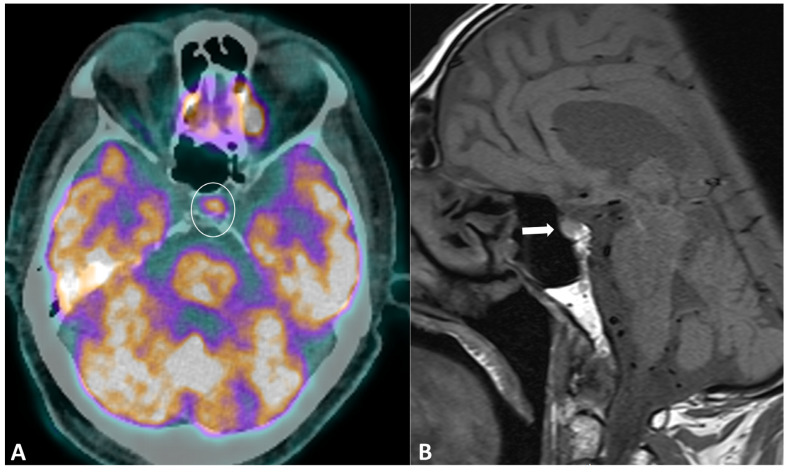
Immune-related hypophysitis in a patient with metastatic lung cancer treated with combined antiPD-1 + antiCTLA-4. A restaging PET/CT (A) showed the appearance of a moderate uptake of the hypophysis (circle). An MRI was then performed, revealing (B) a slight hypophyseal enlargement with homogeneous enhancement (arrow), without significative pituitary stalk thickening noroptic chiasm compression. The hormonal tests were unremarkable, and thus compatible with a grade-1 hypophysitis. ICI treatment was temporally discontinued to ensure the regression of the metabolic activity and the absence of delayed clinical or biological signs, then rechallenged with no recurrence of the hypophysitis.
